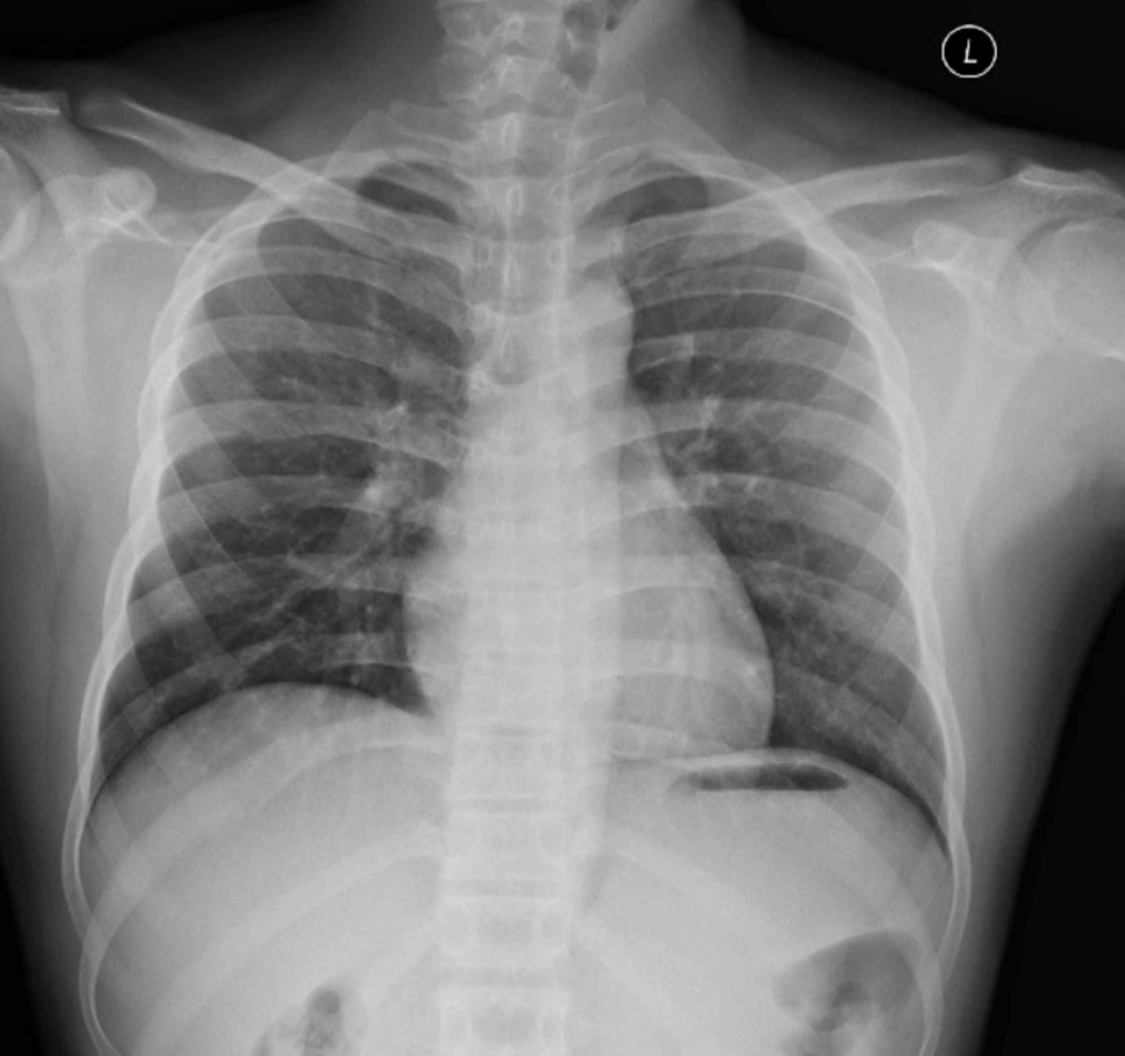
X-ray chest showing complete resolution of pneumomediastinum and subcutaneous emphysema
